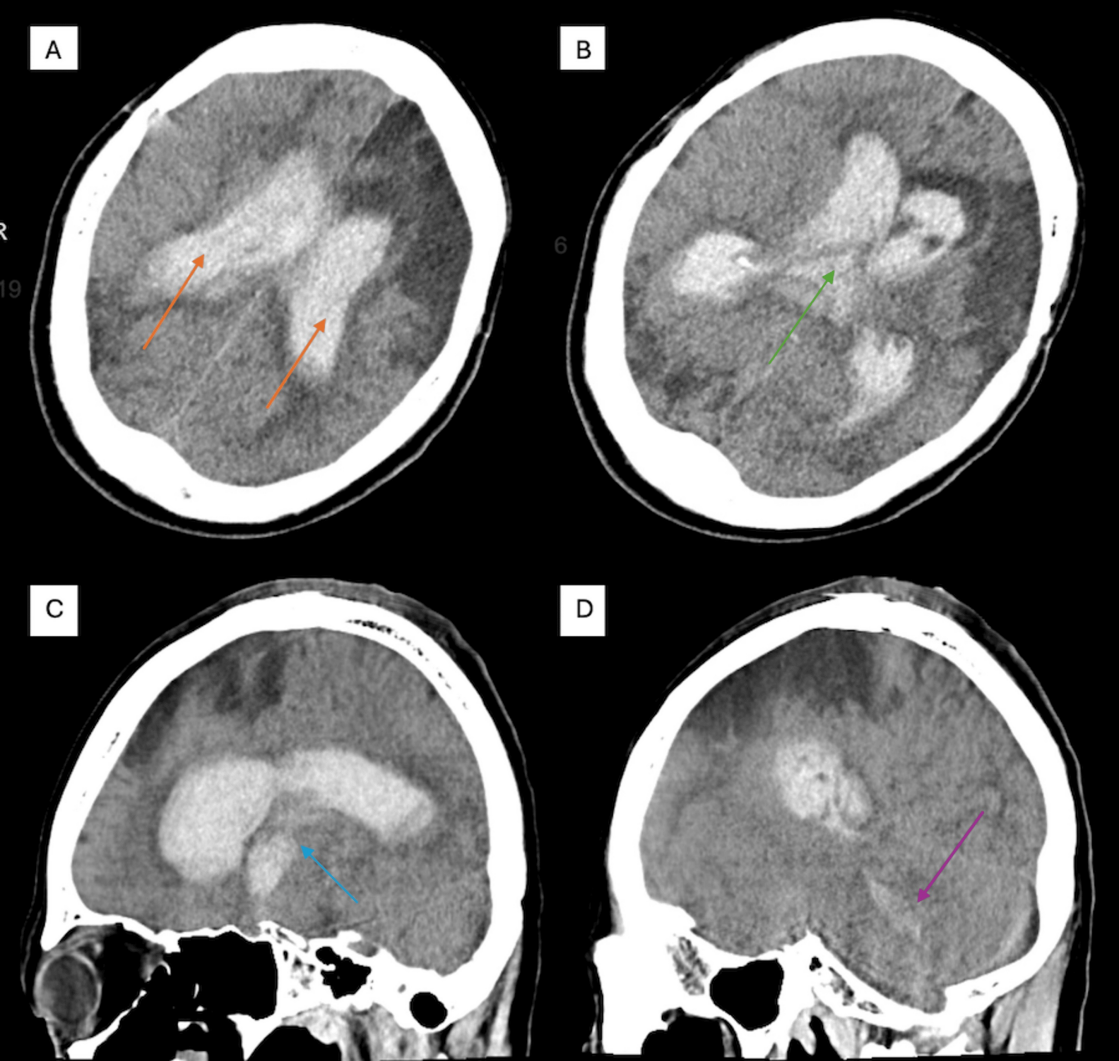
Initial CT head without contrast (A) large intraventricular hemorrhage occupying the bilateral lateral ventricles (orange arrows); (B-D) blood products in the third ventricle (green arrow), cerebral aqueduct (blue arrow), and cisterna magna (purple arrow) CT: computed tomography
